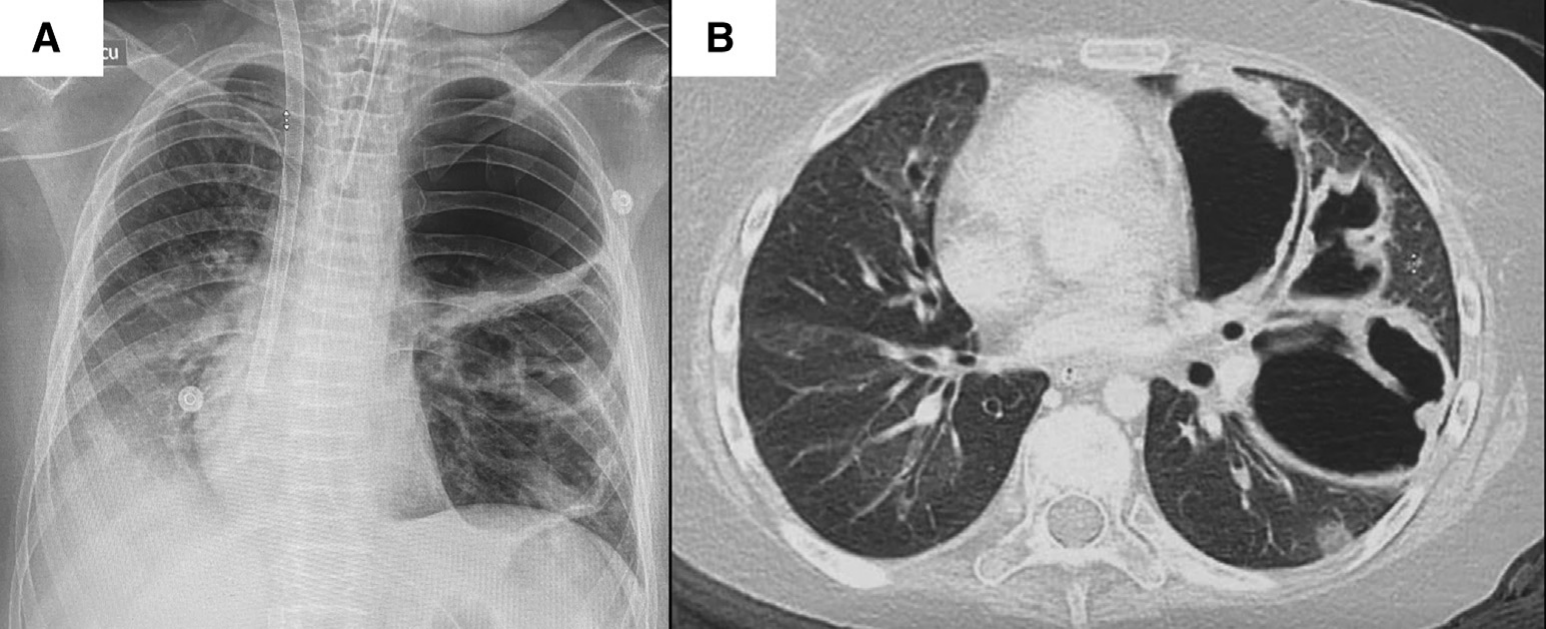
Preoperative radiograph and computed tomography scan. A, Preoperative radiograph shows large cavitary lesion at left upper lung zone, mediastinal shift to the right and peripheral VV ECMO cannulas. B, Preoperative computed tomography shows bronchiectasis throughout the lung, mosaic attenuation of the lung parenchyma, and large cavitary lesion at left upper lobe.
